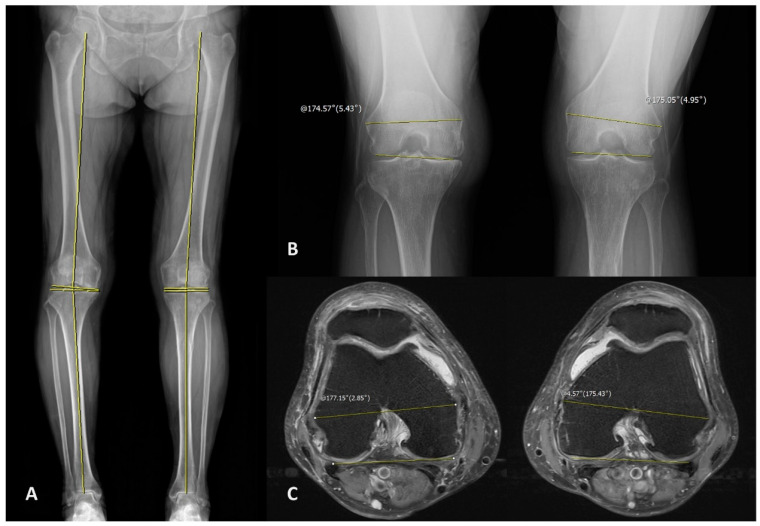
(A) The X-ray image with the reference line drawn for measuring the radiologic makers in the standing lower extremity scanogram. (B) The X-ray image with the reference line drawn for measuring the mid-flexion condylar axis (MFCA)/transepicondylar axis (TEA) in Rosenberg view. A positive value is assigned when the medial side is open. (C) The magnetic resonance imaging (MRI) axial image with the reference line drawn for measuring the posterior condylar axis (PCA)/TEA. A positive value is assigned when the medial side is open.
